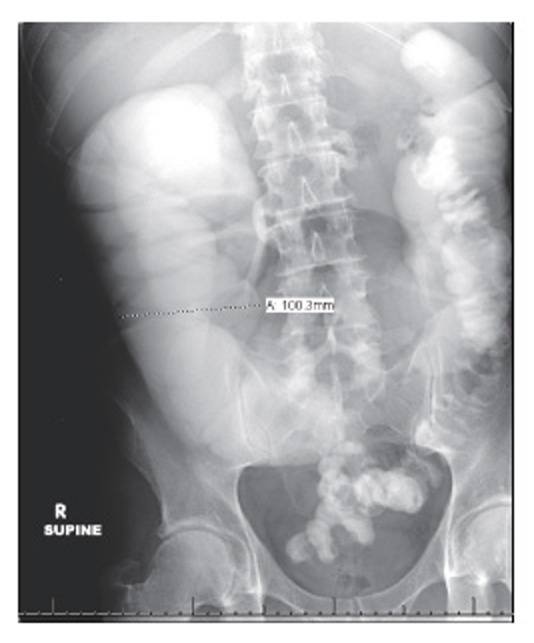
Follow-up abdominal roentgenogram after barium enema revealed retained contrast with increasingly dilated proximal colon to 10 cm.
